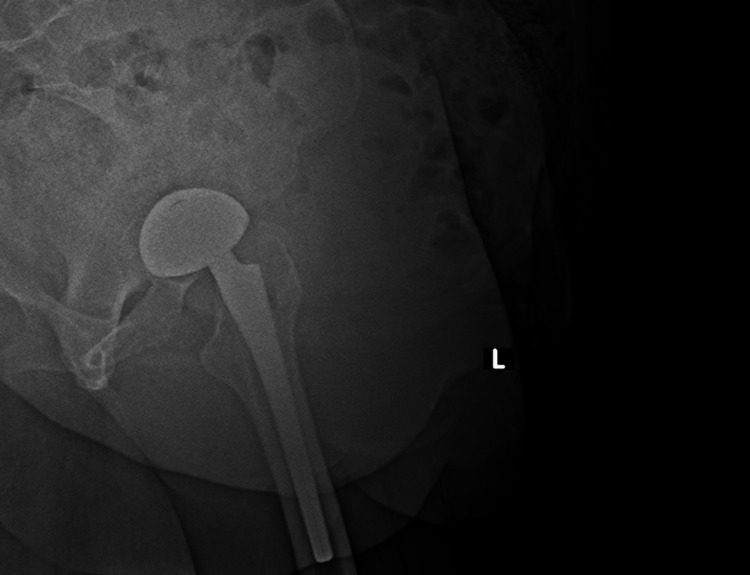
The lateral radiograph of the left hip joint (two years after left total hip arthroplasty) showing displacement of femoral head prosthesis with respect to the acetabular cup.
